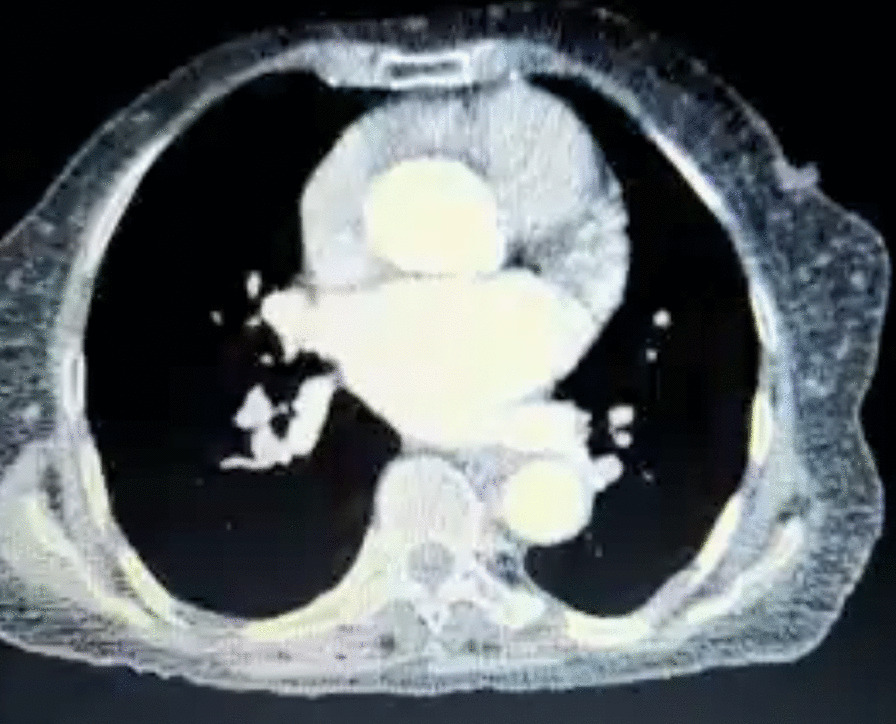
Postoperative computed tomography (CT) scan of a 66-year-old female, after left thoracotomy due to large solitary fibrous tumor of the mediastinum, demonstrating no significant findings
